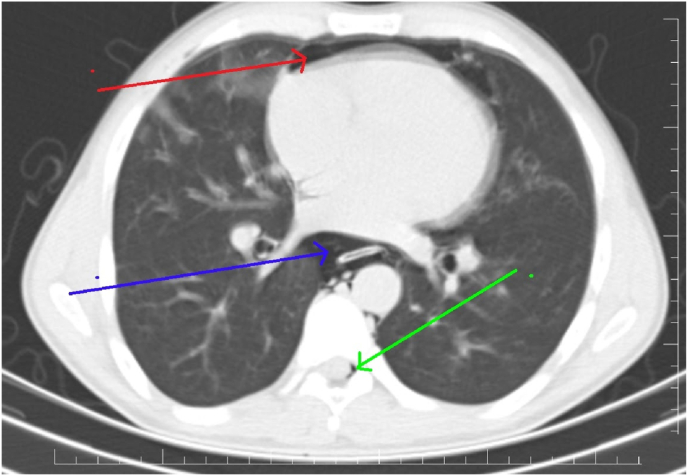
CT scan showing pneumomediastinum (blue arrow), pneumopericardium (red arrows) and pneumorrachis (green arrow). (For interpretation of the references to colour in this figure legend, the reader is referred to the Web version of this article.)
